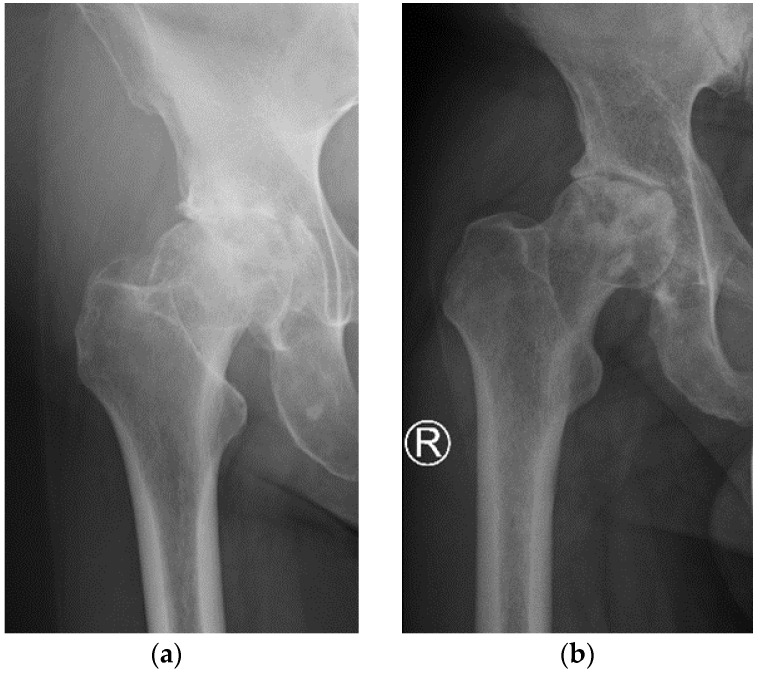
Pre-operative AP X-Ray right hip joints of two patients: (a) patient 2; (b) patient 4.
